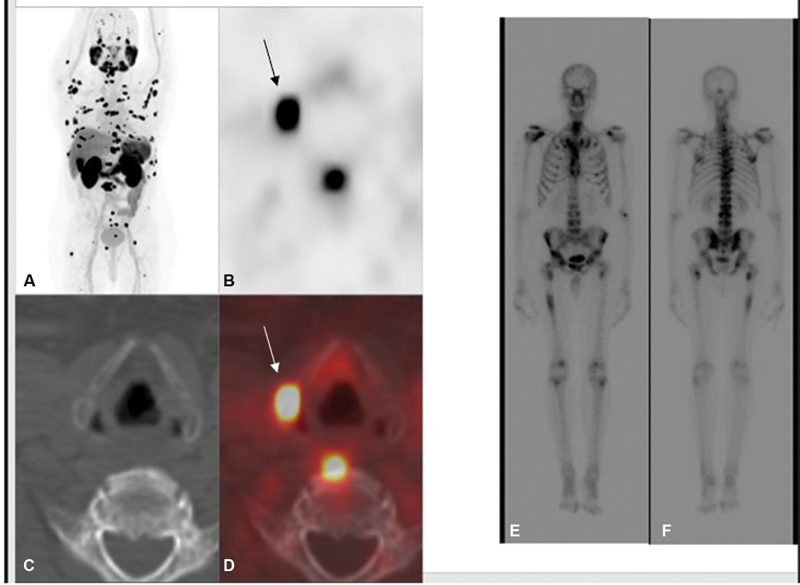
Coronal MIP 18F PSMA PET CT of the third patient (
